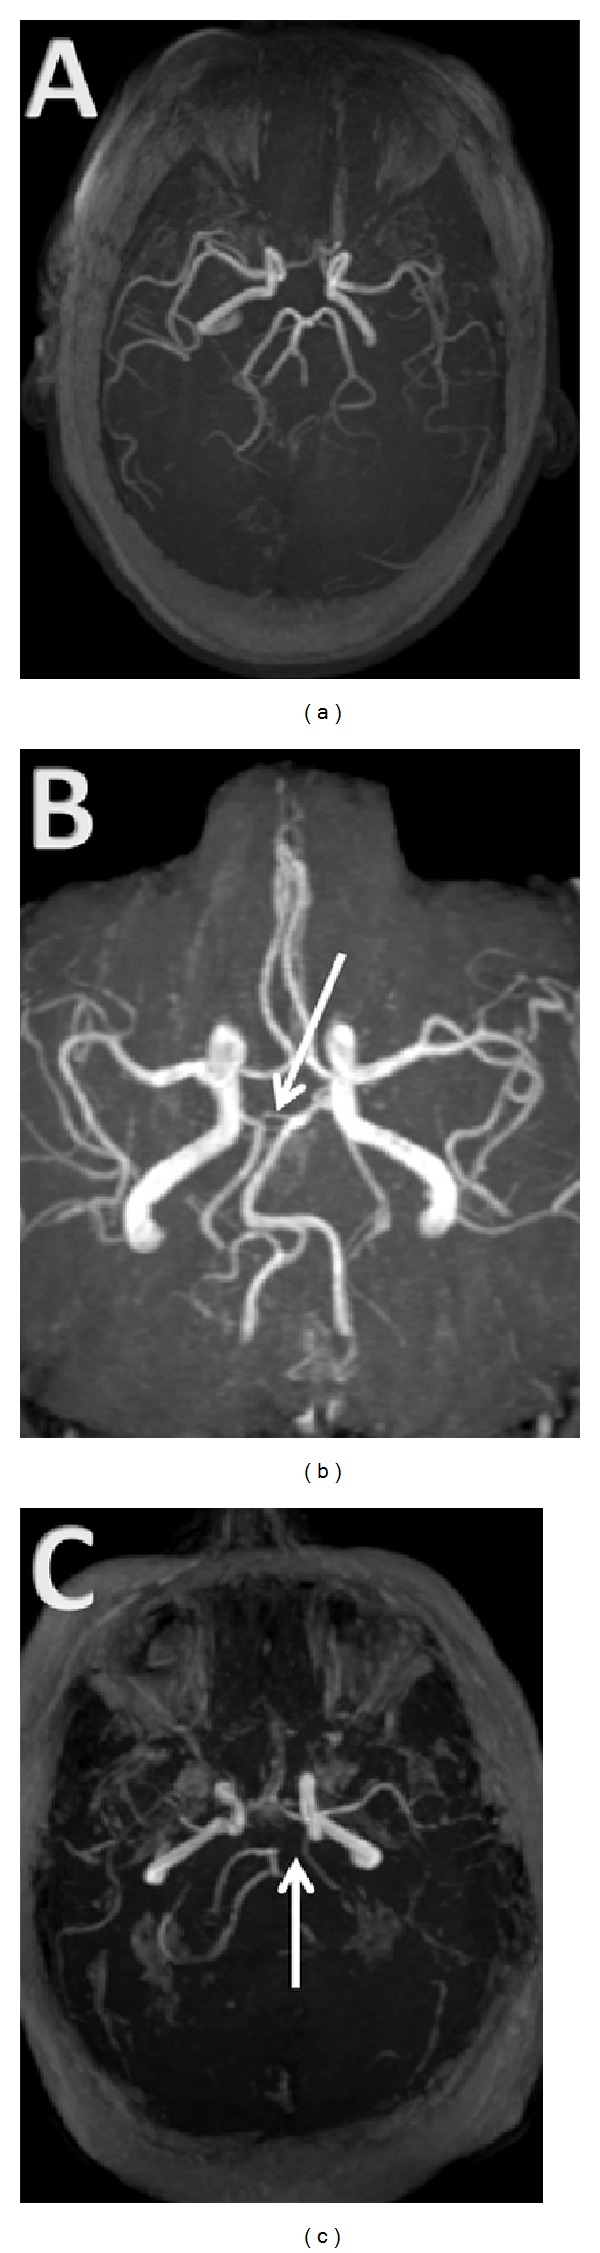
MRI TOF demonstrating a complete fetal PCA (cfPCA) and partial fetal PCA (pfPCA). (a) Typical pattern of PCAs as terminus of basilar artery. (b) Partial fPCA on the right side. The arrow shows an atretic communication between the basilar artery and the posterior communicating artery forming the PCA. (c) Complete fPCA on the left side. The arrow shows lack of communication between the basilar artery and the PCA.
